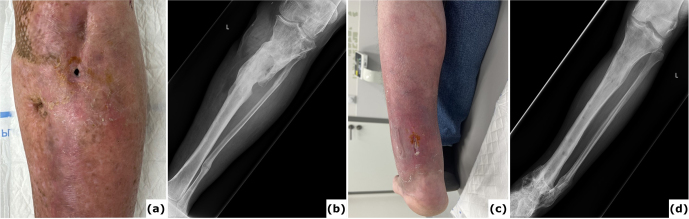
Chronic infection (osteomyelitis) following extended external fixation. (a) Clinical image presenting a fistula with purulent fluid drainage in a 64-year-old patient after multiple surgical procedures for fracture-related infection (FRI) of the proximal tibia with non-union. (b) Anteroposterior radiograph of the tibia showing consolidation of the proximal tibia fracture; the external fixation time (EFT) was 16 months. (c) Clinical image depicting a chronic wound on the posterior aspect of the ankle in a 54-year-old patient after several surgical interventions for FRI of the distal tibia. Bone transport was performed as treatment, with an EFT of 12 months. At referral, the patient had local and systemic signs of infection and was prescribed oral antibiotics. These episodes have recurred intermittently over 3 years following removal of the external fixator. (d) Anteroposterior radiograph of the tibia demonstrating consolidation at all sites.
